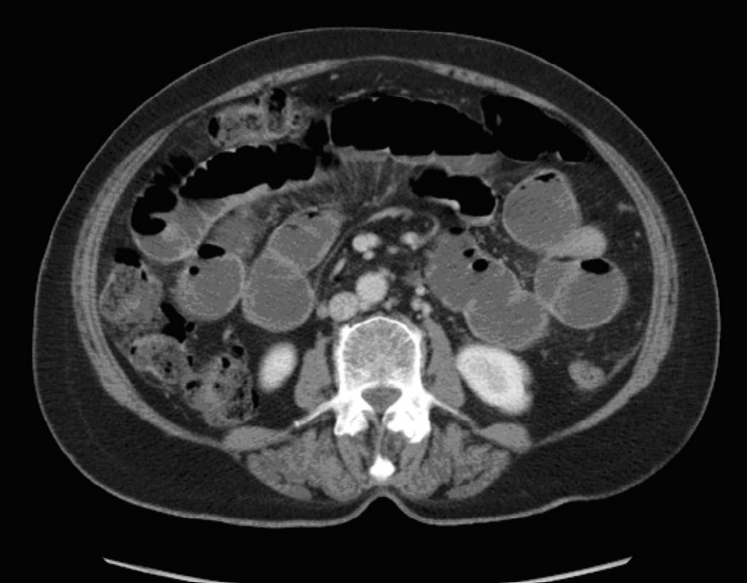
Emergency CT scan showing small bowel dilation with a point of abrupt change in calibre and angulation in the distal jejunal loop. The small bowel distal to this point was collapsed.
